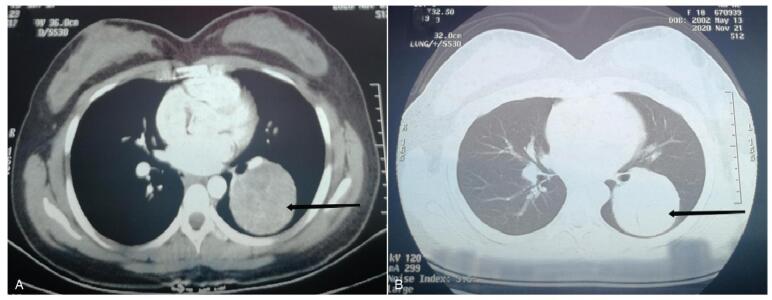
Figure 1. Technique: Chest enhanced CT. (A) and (B) showed a mass in the left lower lobe, about 6 cm in diameter, with clear boundary and less uniform density. CT=computed tomography.
